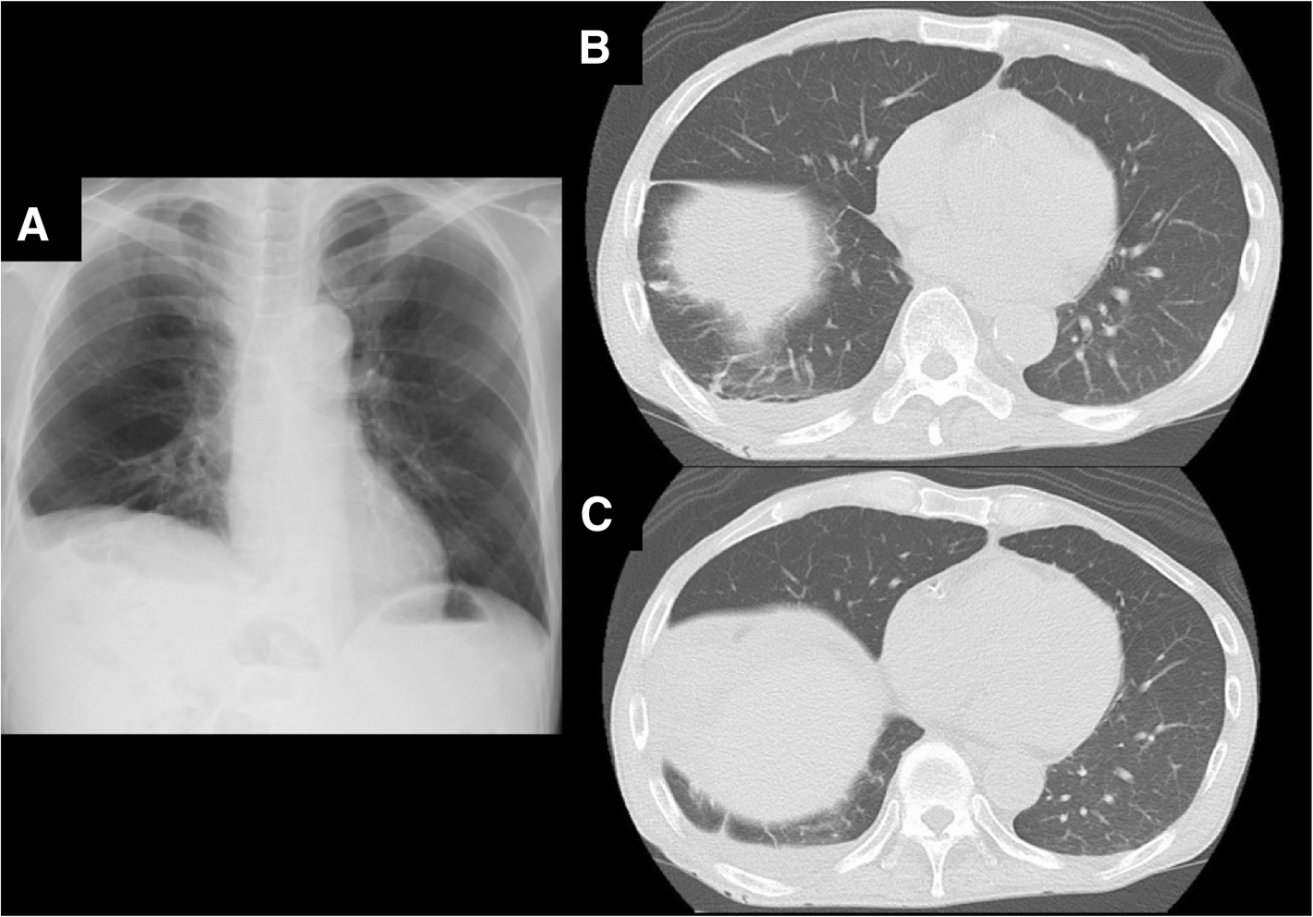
Postoperative chest X-ray and computed tomographic (CT) images taken at 3 months after surgery. a Chest X-ray showed the expansion of the right lung. b, c CT images showed that no cyst existed in the bottom of the right lung
